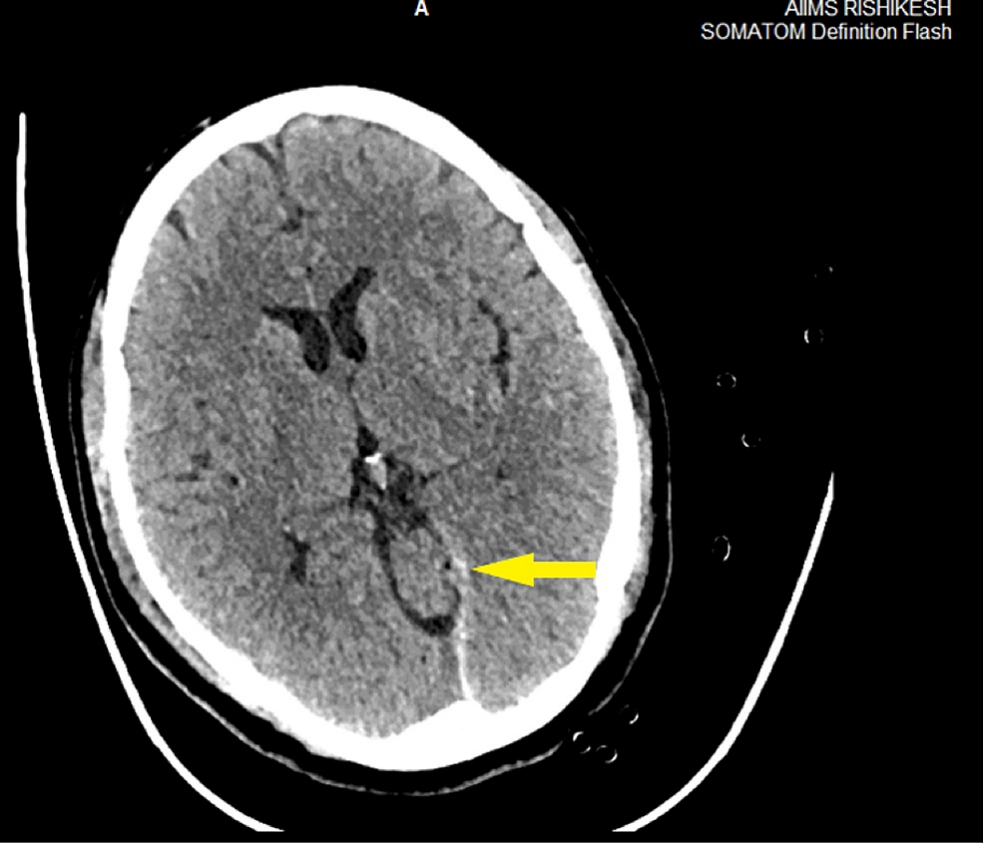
Non-contrast CT scan of the patient showing subarachnoid hemorrhage in the left Sylvian fissure.
